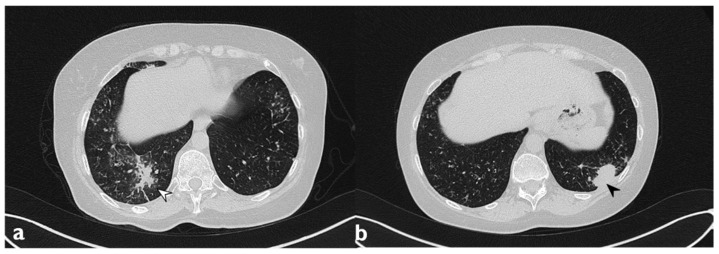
Migratory infiltrates. On the left (a): baseline HRCT; subpleural consolidation in the right lower lobe (white arrowhead). On the right (b): HRCT after 13 months of steroid therapy; the consolidations have disappeared in the first site, appearing in the left lower lobe (arrowhead).
